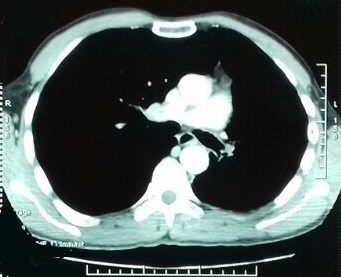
Mediastinal section thoracic CT scan: reduction in the diameter of the left pulmonary artery with poorly developed collateral bronchi
